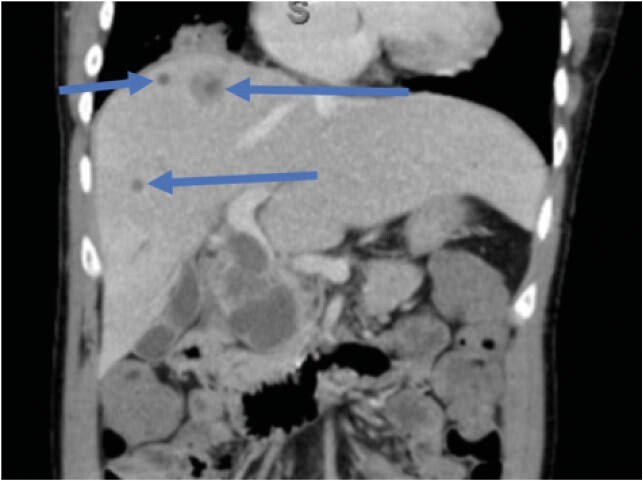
Serial CT scan (coronal view) of abdomen demonstrating multiple hepatic abscesses (blue arrows)
